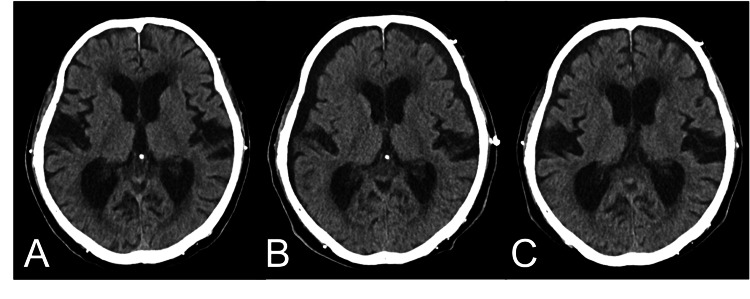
Axial head CT scan at (A) day 1 show no evidence of intracranial hypotension, (B) day 7 reveal subdural hygroma and narrow ventricular, suggesting intracranial hypotension, and (C) day 14 show improvement.
